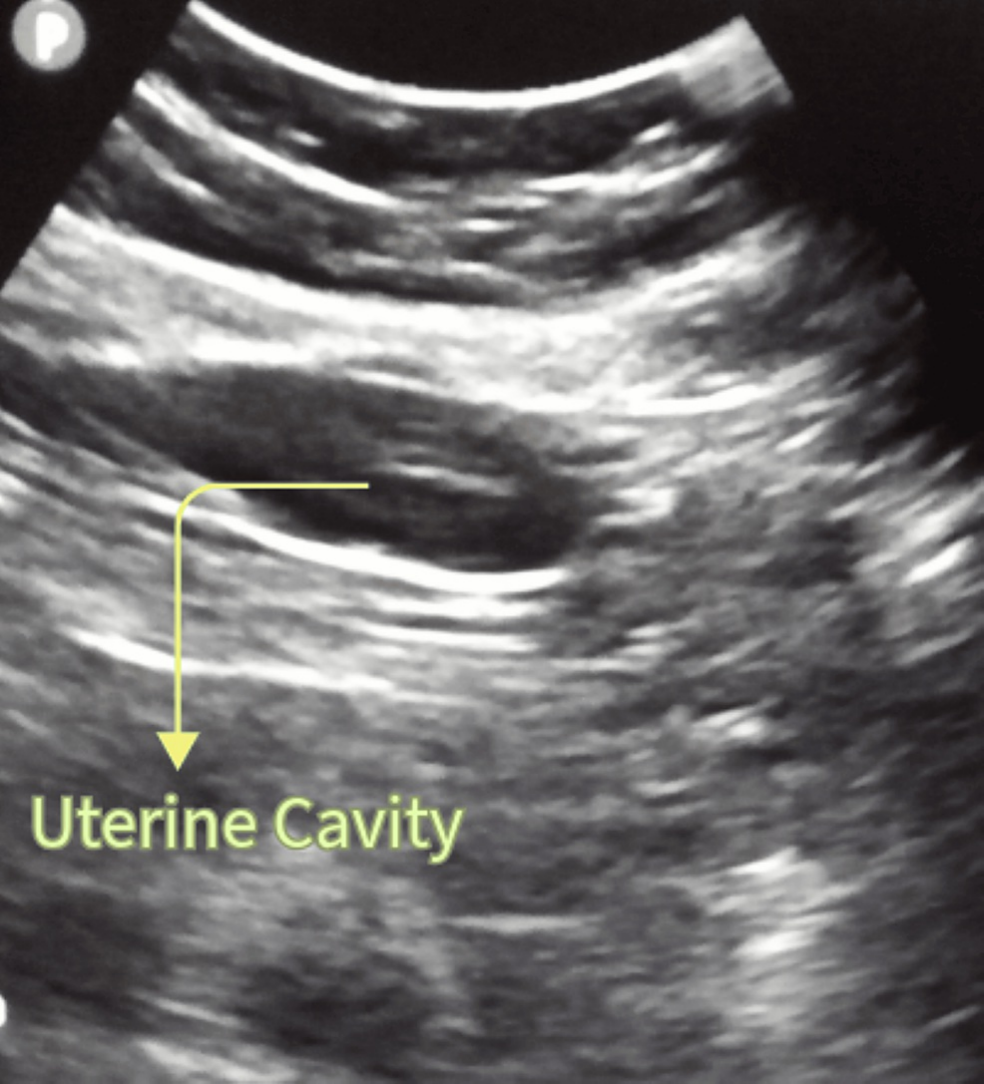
A TVS of the patient's uterine cavity and endometrium on day 17 of the menstrual cycle TVS: transvaginal sonography
